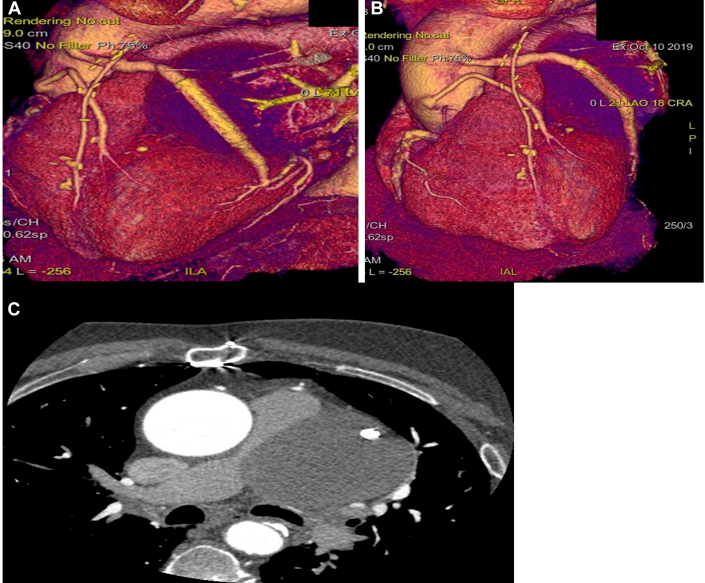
Follow-Up Computed Tomography (A to C) Postprocedural computed tomography results showing thrombosed pseudoaneurysm.
